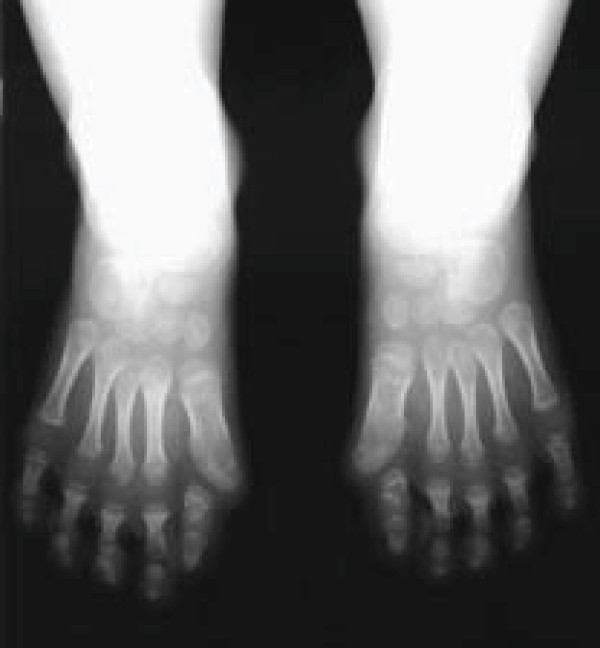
Anteroposterior radiograph of the feet of a three-year old child showing symmetrical first toe malformations.  Reprinted from reference 14. Authors retain copyright.
